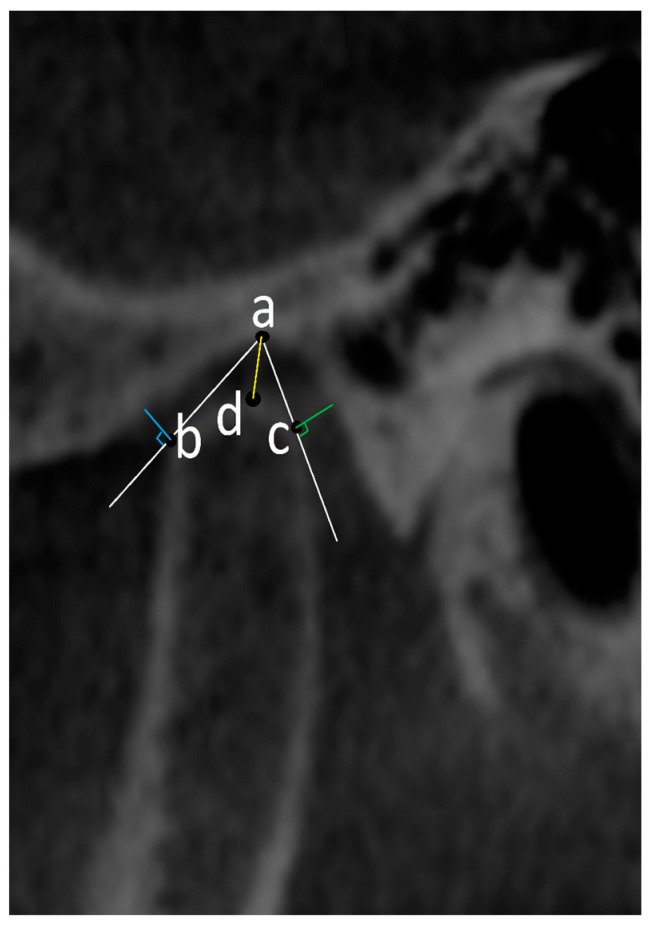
Oblique sagittal reformatted CBCT image showing the measurement of the anterior joint space (AJS), posterior joint space (PJS), and superior joint space (SJS). The measurements were carried out on the image in the sagittal plane corresponding to the middle point of the condyle on the axial plane and coronal planes on the slices with the largest condylar dimensions. Point (a) is drawn at the uppermost point of the mandibular fossa from which two tangents (white lines) are drawn on the anterior (b) and posterior (c) margins of the condylar head and using a line perpendicular to these tangents, the AJS (blue line) and PJS (green line) are measured. Another line joining point (a) and the most superior part of the condyle (d) was used to measure the SJS (yellow line).
